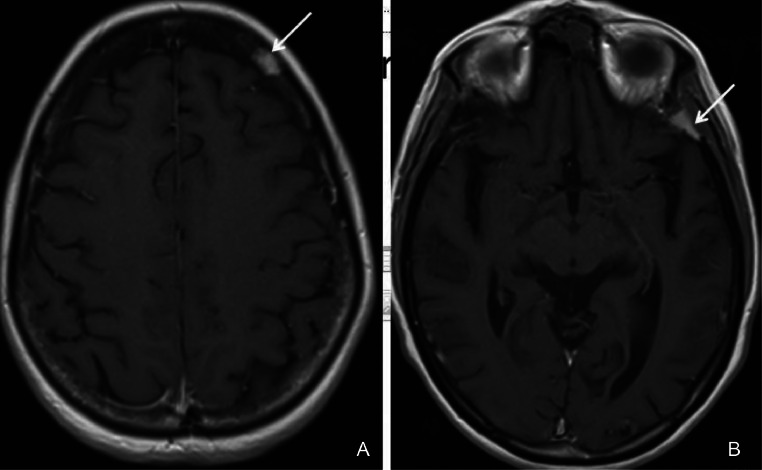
Brain MRI: Axial T1 sequence showing enhanced bone lesions after Gadolinium injection, frontal (A) and left temporal (B).
